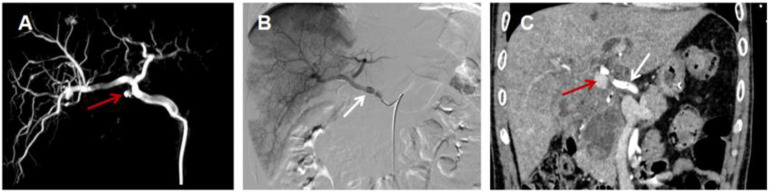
(A) Celiac arteriography showing a proper hepatic artery pseudoaneurysm (red arrow); (B) Postoperative angiography after proper hepatic artery covered stent placement (white arrow) showing normal arterial course, patent distal flow, and no contrast leakage and (C) Abdominal CTA after covered stent placement (white arrow) showing contrast leakage (red arrow).
